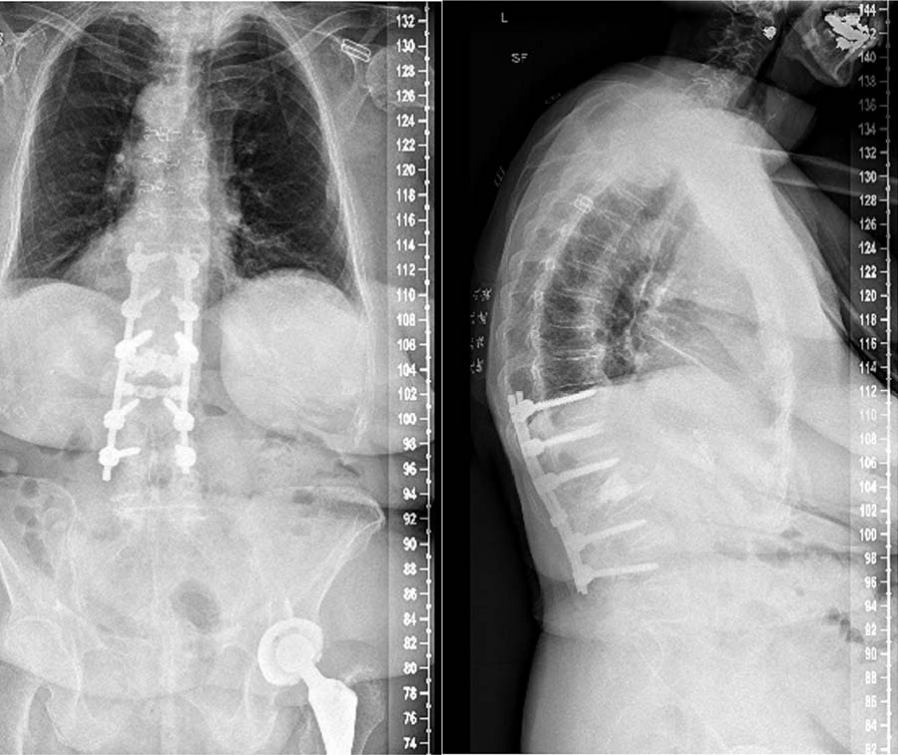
Anterior–posterior and lateral radiographs of the patient’s spine postoperatively, following L1-L2 laminectomy and T11-L4 posterior fusion.
